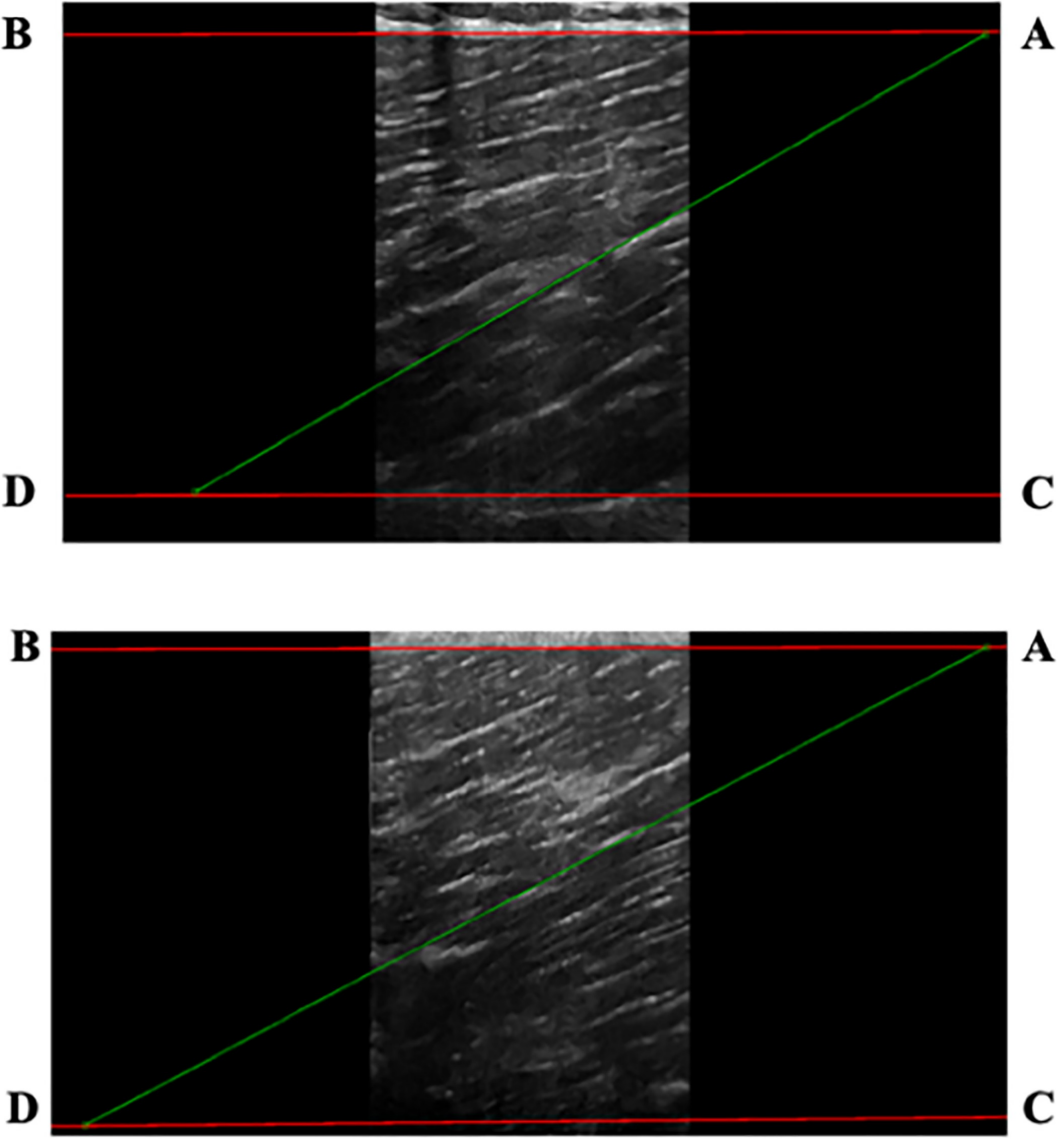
Two-dimensional ultrasound image of the pre-post intervention of Biceps Femoris long head (BFlh). In order to measure FL and PA, a line was placed along the length of a fascicle, which joined superficial aponeurosis (A-B line) and intermediate aponeurosis (C-D line). The FL was calculated as the length of this line. The PA was calculated as the angle between lines CD and DA.
